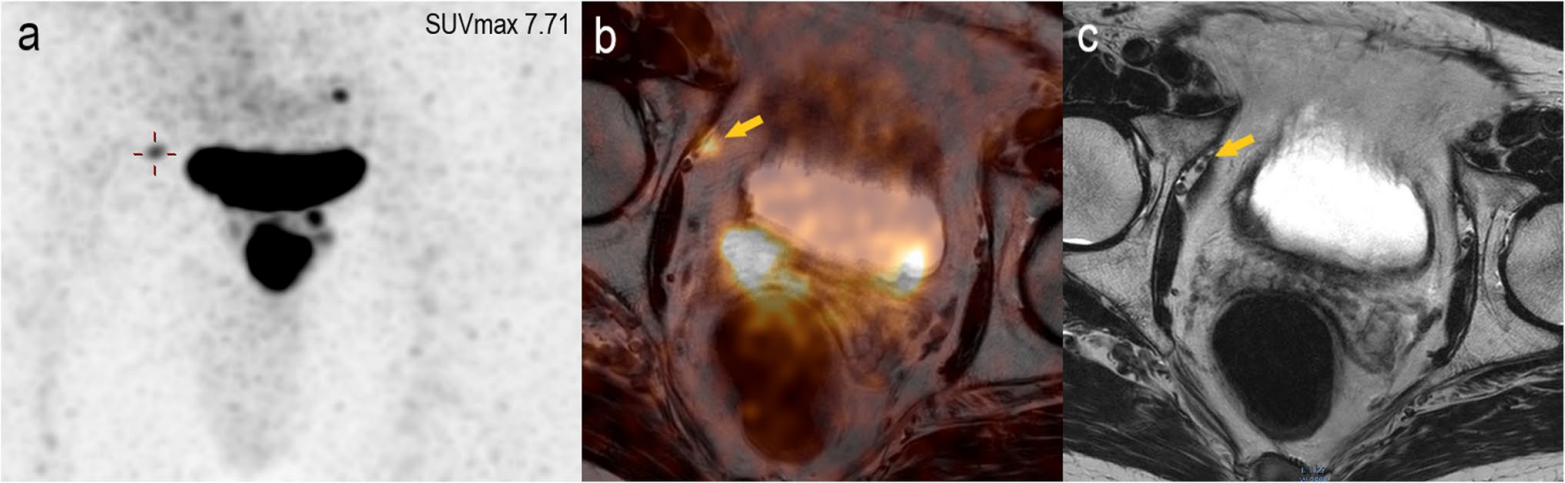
The potential of [^68^ Ga]Ga-PSMA-11 PET in the detection of small LN clearly superior to mpMRI. A 71-year-old high-risk prostate cancer patient, Gleason score 6 and tPSA of 20.96 ng/ml showed suspicious uptake in a pelvic LN in the maximum intensity projection image (a) and the fused PET/CT image (b). However, it did not fulfill the criteria for malignancy on T2-weighted MR imaging (c).
